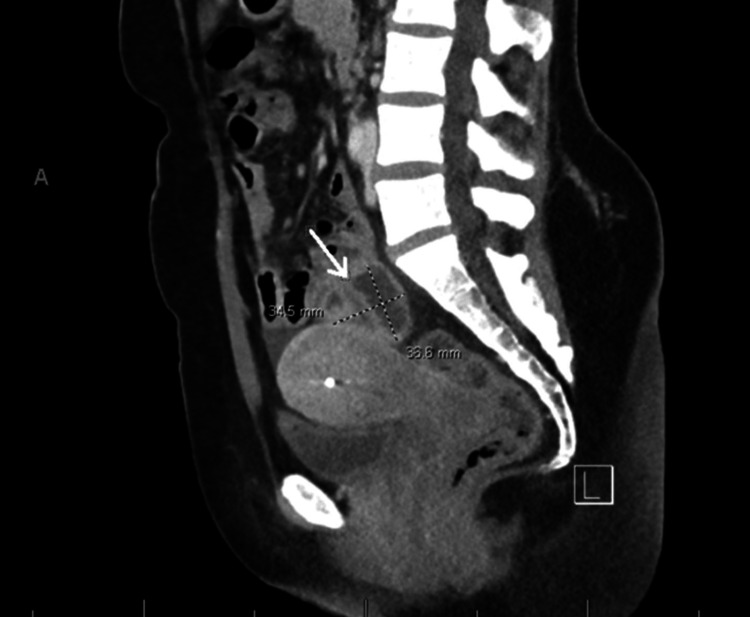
Longitudinal CT of the abdomen and pelvis demonstrating a large retrouterine mass (arrow) measuring 34.5 × 36.6 cm. The bright white spot within the uterus represents an intrauterine device (IUD).
